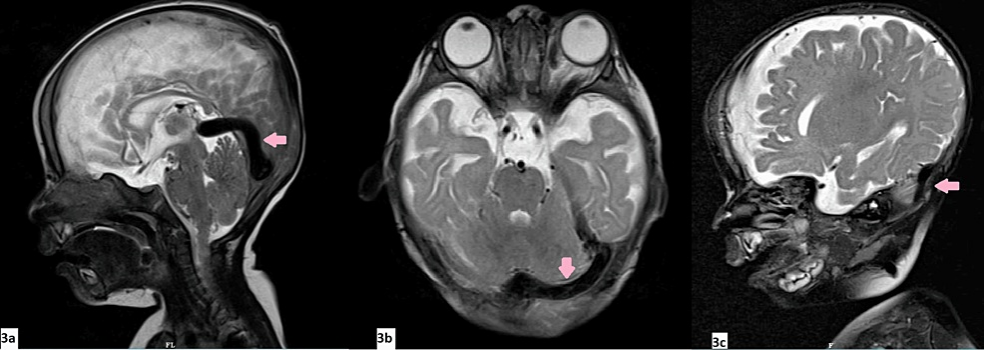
Sagittal (3a and 3c) and axial (3b) T2-weighted MR images showed dilatation of the straight sinus (3a), left transverse sinus (3b), and left sigmoid sinus (3c)
